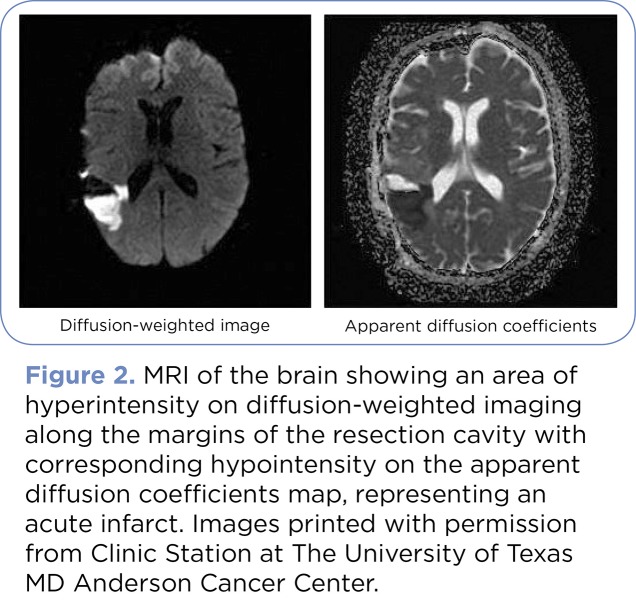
Figure 2. MRI of the brain showing an area of hyperintensity on
